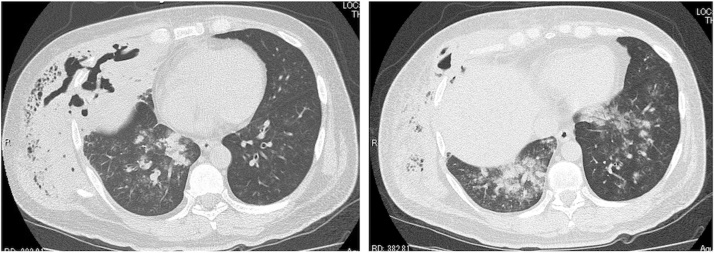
Chest computed tomography (CT) on day 1.
